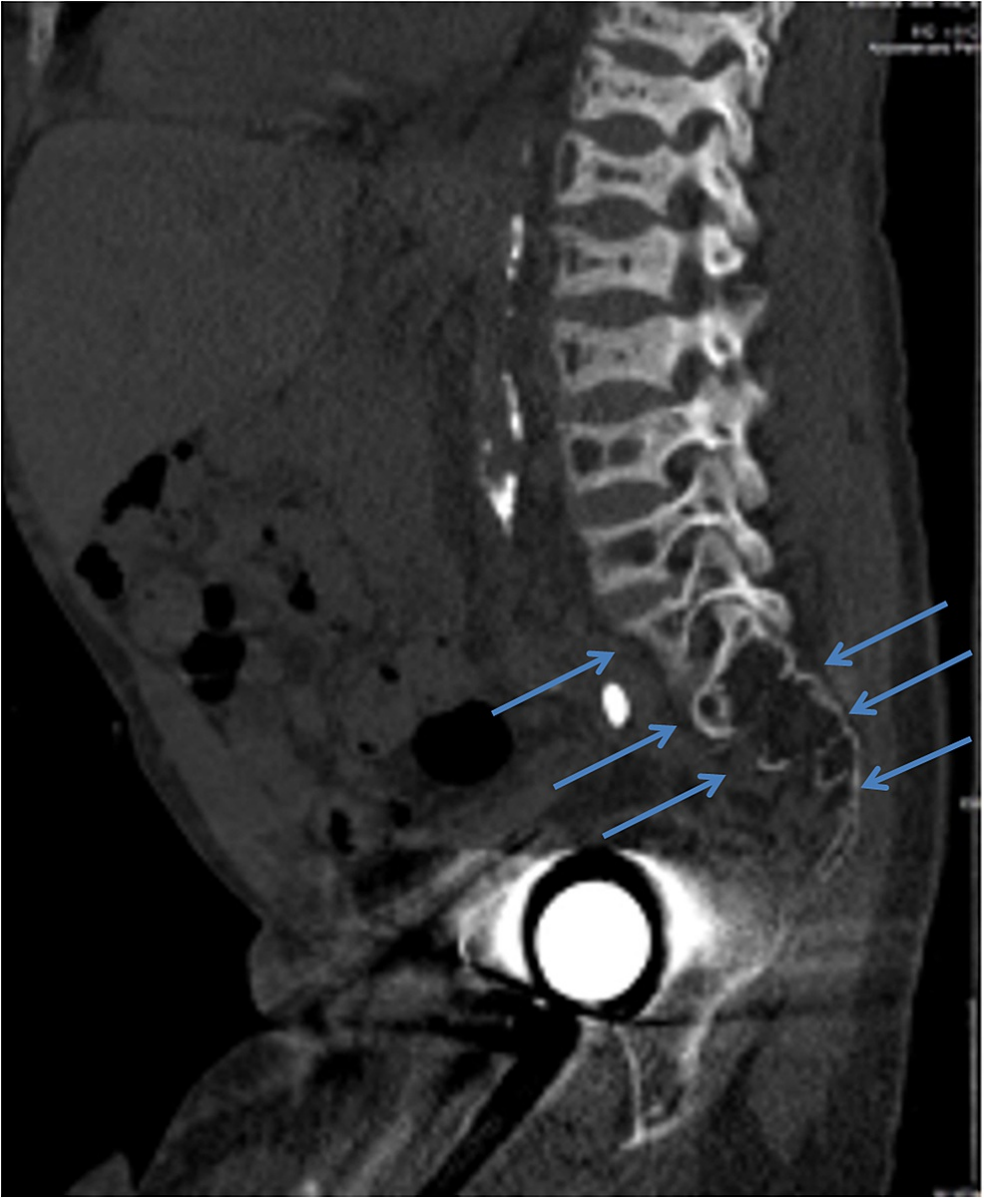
CT of the lumbar spine with marked height loss of lumbar vertebral bodies (arrows) CT: computed tomography
